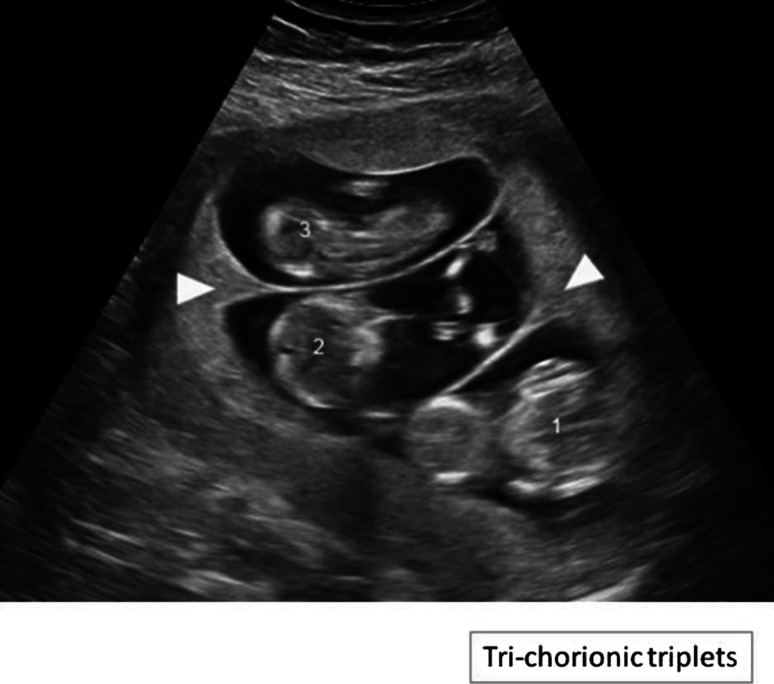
Ultrasound picture of a trichorionic trio at 12 weeks gestational age. These three fetuses do not share their placentas. This trio can be trizygotic, dizygotic (one identical duo), or monozygotic. Numbers indicate the three fetuses. (Used with permission from Lamb et al. 2012)
